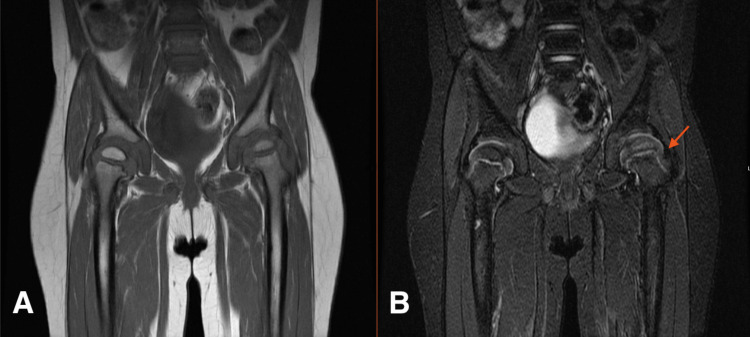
MRI pelvis body T1 (A) and T2 (B). Symmetric bilateral capital femoral and greater trochanter physis signal intensities with no evident SCFE. However, mild diffuse increased T2 signal intensity of the left femoral head (red arrow) with normal height is seen, differentials may include subtle edema for early AVN. SCFE, slipped capital femoral epiphyses; AVN, avascular necrosis
